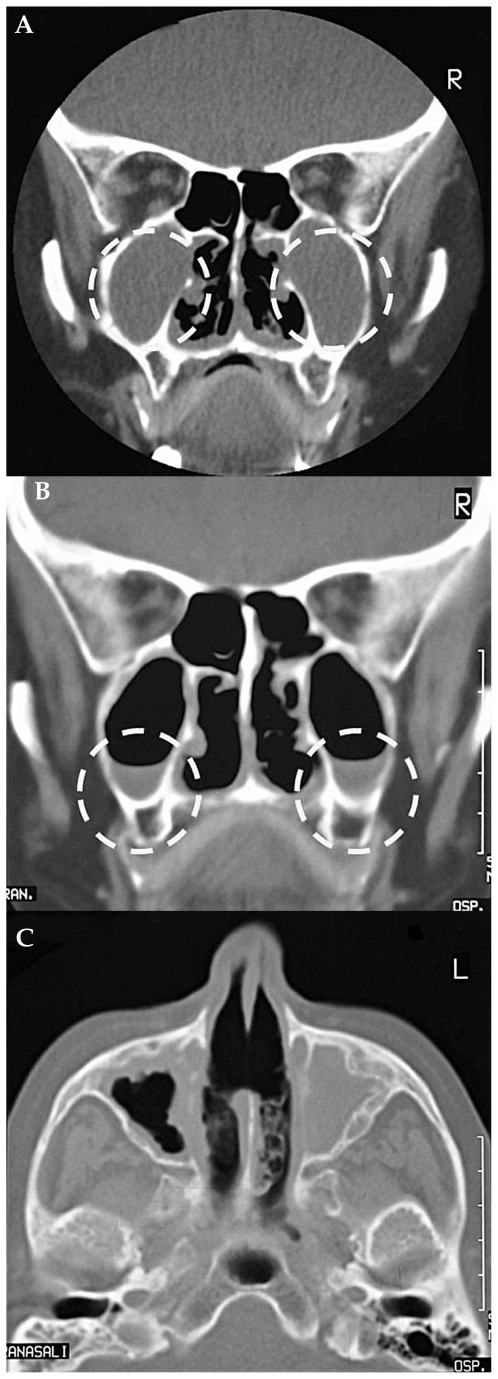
Wegener’s granulomatosis (CT scan without contrast): the maxillary sinuses are filled with granulomatous tissue (A); the same lesions, after treatment, show reduction in granulomatous invasion (B); perforation of the nasal septum (C).
